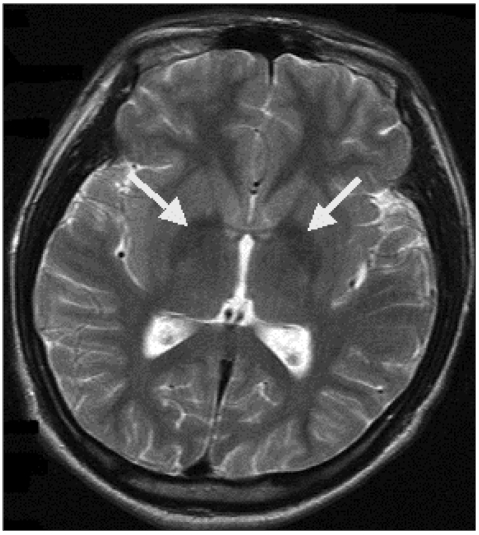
Magnetic resonance imaging. T2-weighted axial images showing areas of slightly decreased signal intensity in the globus pallidus bilaterally.
